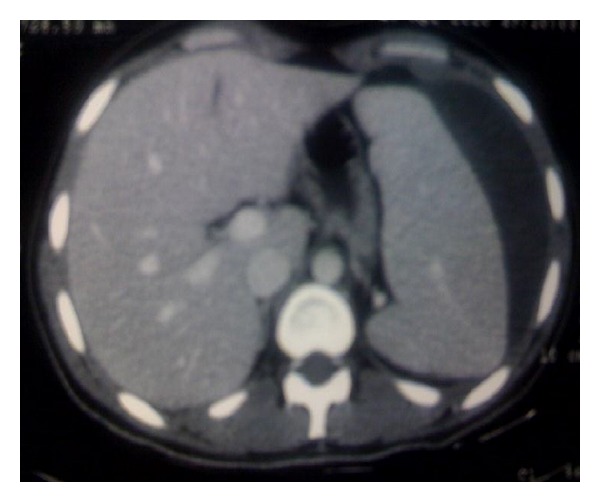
CT, two weeks after patient's admission, showed the same splenic hematoma.
